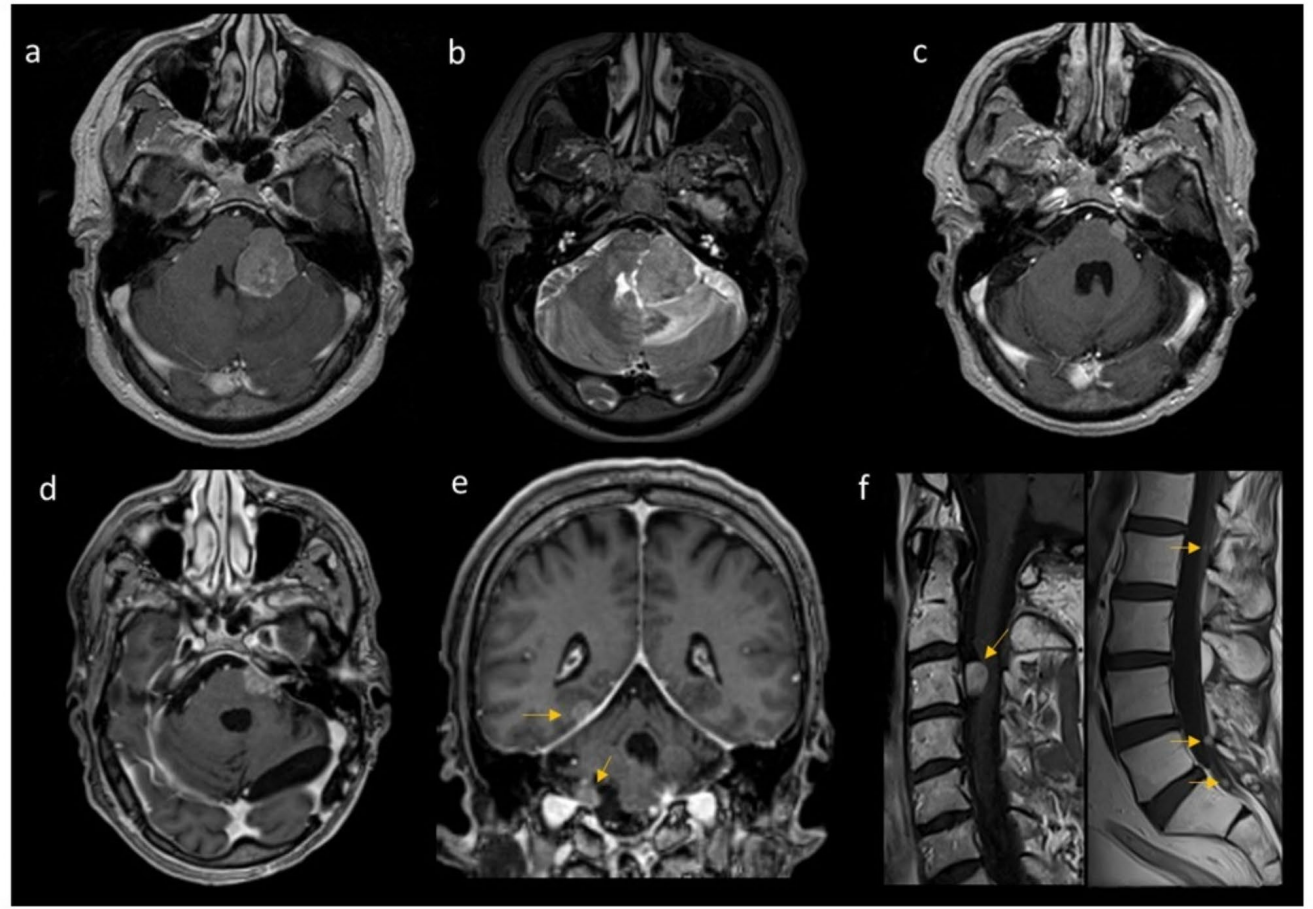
Patient 13; a 46-year-old man bearing an extra-axial cerebellopontine angle lesion with mass effect and reactive edema on cerebellar hemisphere, fourth ventricle and pons. First pre-surgical Magnetic Resonance (MR) (a, b); pre-radiotherapy MR 2 years later, at Disease Progression (c); last follow-up MR, 7 years from first surgery (d-f), showing supra-, infra-tentorial and spinal nodular dissemination (arrows). Brain (a-e) and spinal (f) T1-weighted images post- contrast agent administration (a, c-f) and T2-weighted images (b)
